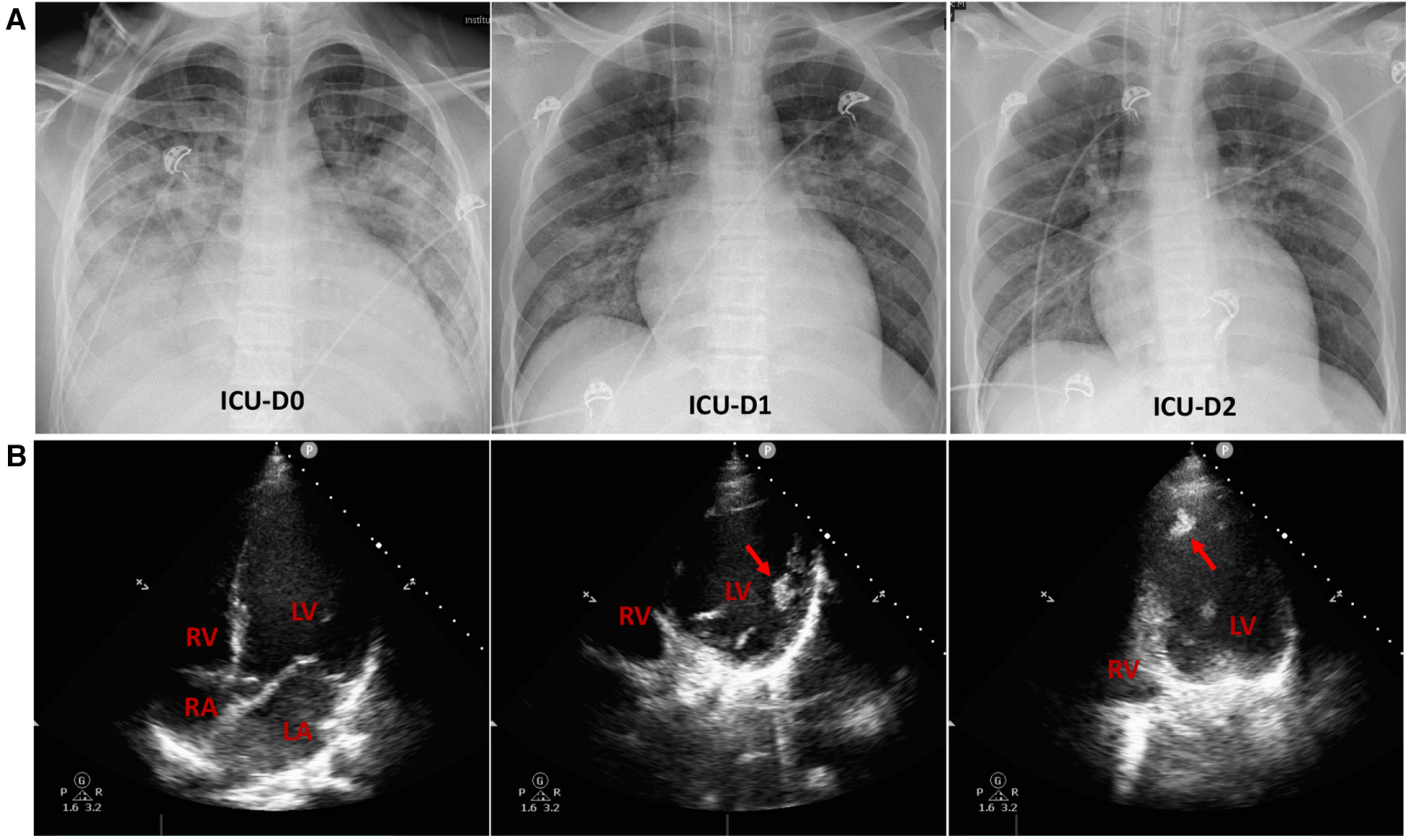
Chest radiographs and echocardiograms in the intensive care unit (ICU). (A) Chest radiographs on the initial three days of ICU admission. Lung edema exhibited a rapid amelioration. (B) On ICU admission, the apical 4-chamber views of the echocardiography indicated enlarged left atrium and ventricle, along with apical thrombi (indicated by red arrows) in the dilated left ventricle. RV, right ventricle; RA, right atrium; LV, left ventricle; LA, left atrium.
